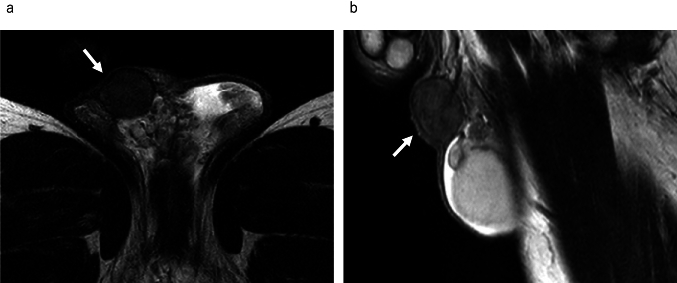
Scrotal leiomyoma in a 40-year-old-male with a scrotal mass recognizing for more than 10 years. a Axial T2-weighted MR image shows a homogeneous hypointense mass (white arrow) attached to the Dartos fascia in the right scrotum near the right spermatic cord. b Sagittal T2-weighted MR image shows a hypointense mass (white arrow), which is located in the right scrotum above the right testis
